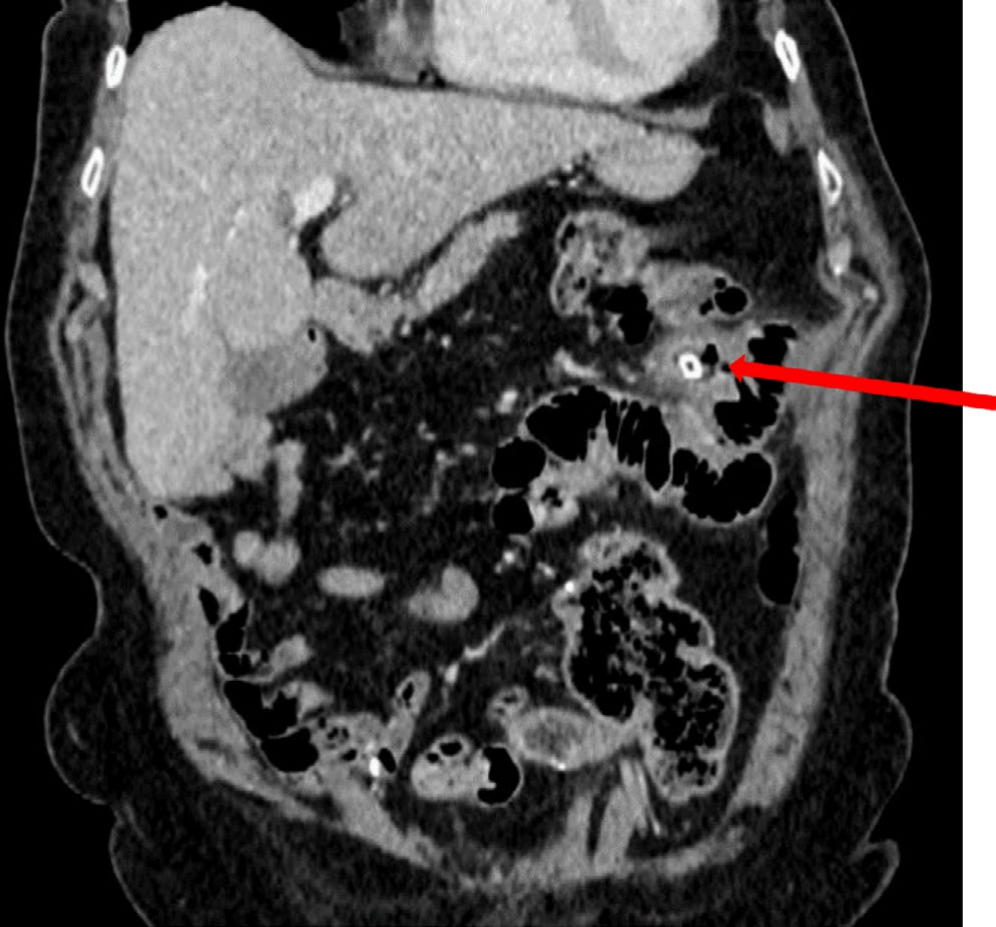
Coronal reconstruction from the follow‐up CT scan performed 2 weeks after the initial study. The tubular hyperdense foreign body (red arrow) remains in a fixed position within a jejunal diverticulum, consistent with impaction. Compared to the initial scan, there is now localized fat stranding and subtle haziness of the surrounding mesenteric fat, indicative of a developing inflammatory reaction. These findings are suggestive of jejunal diverticulitis secondary to the retained foreign body. Notably, the foreign body tip appears to abut or potentially breach the mucosal surface, raising concern for early transmural involvement. The constellation of persistent foreign body impaction, mucosal irritation, and evolving localized inflammation supports the need for surgical intervention to prevent complications such as perforation or abscess formation.
